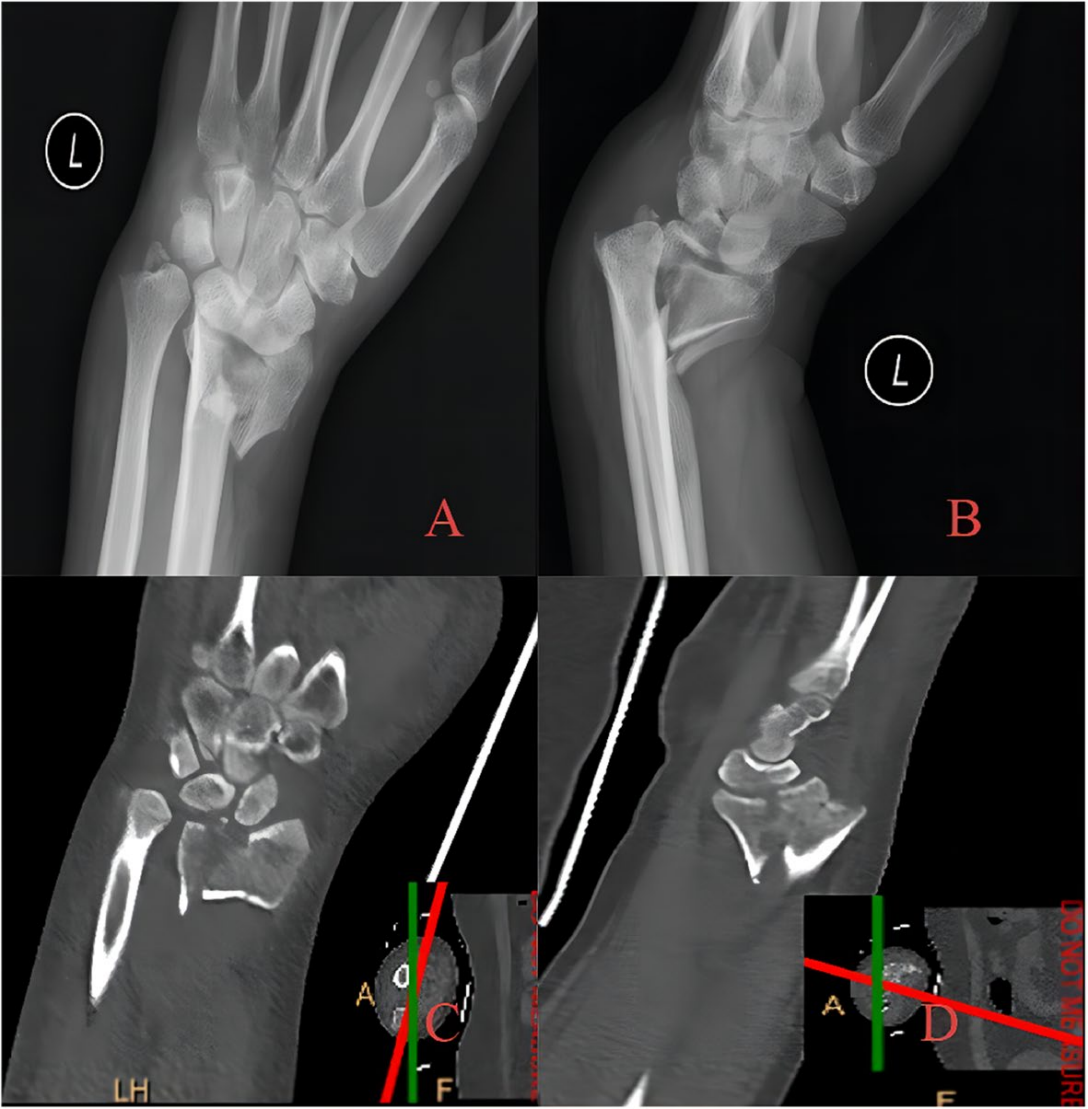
A 27-year-old patient with die-punch DRF. Radiographic examination showed that the lunate surface of the wrist joint was split longitudinally and collapsed downwards, and the fracture fragment was displaced to the palmar side. A AP radiography showed that the epiphysis and articular surface were involved; B Lateral radiography showed fracture; C Axial view of the articular surface of the radius. Fracture involving articular surface of intermediate column and lateral column are noticed; D Coronal plane, collapse of the lunate articular surface is obvious; E Sagittal plane, bone fragment shifted to dorsal side F Three-dimensional reconstruction of CT image
